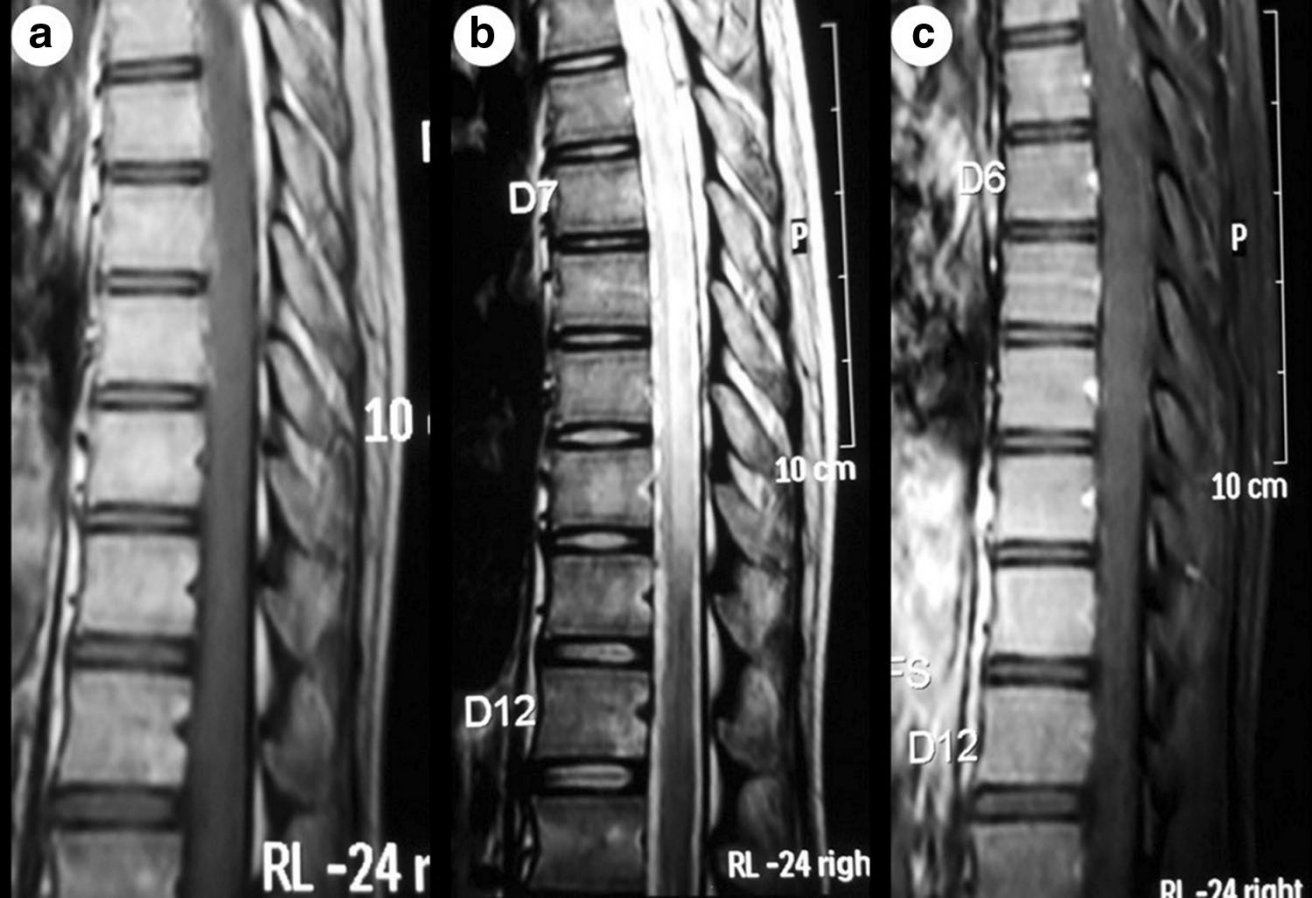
Magnetic resonance imaging (MRI) of thoracic spine (sagittal section) showing contiguous long segment intramedullary lesion which is isointense on T1-weighted (a) and hyperintense on T2-weighted (b) images extending from thoracic vertebral level T2 to T10. There is no cord expansion or contrast enhancement (c)
